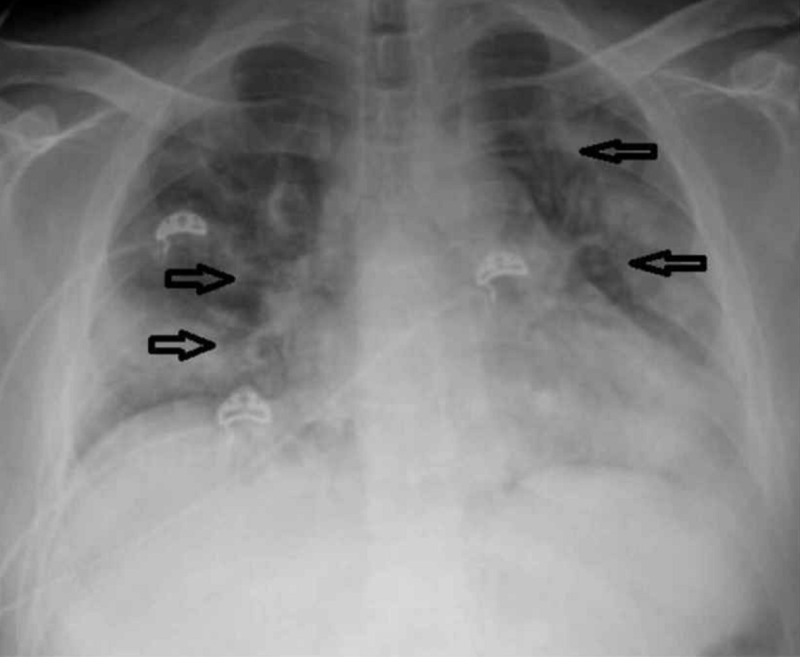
Chest X-ray portable revealed cardiomegaly with bilateral lung infiltrates
